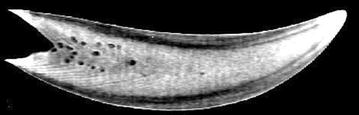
CT image of tooth B using Biograph 64 and the scan- and reconstruction parameters in the CT method details. The slice is similar (but not exactly the same) as in Fig. 2. This image has smaller slice thickness and the filter was not as smooth as in Fig. 2. Thus, this image has more noise but higher spatial resolution, which improves visualization of the fine V-shaped pattern. Still only parts of the V-shaped structures can be visualized and the total number of layers cannot be determined from these images
